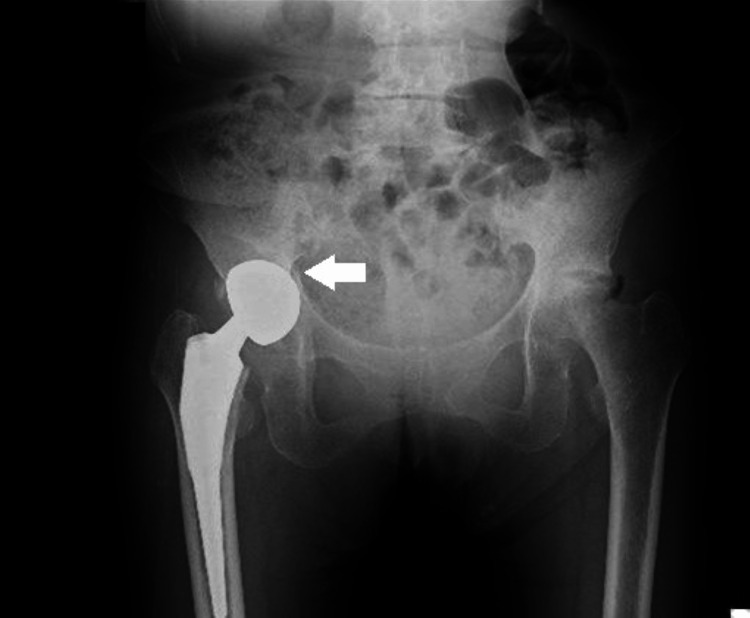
Plain radiograph of the pelvis at admission (anteroposterior view). A right acetabular fracture was identified (arrow), with no obvious loosening of the femoral stem.
